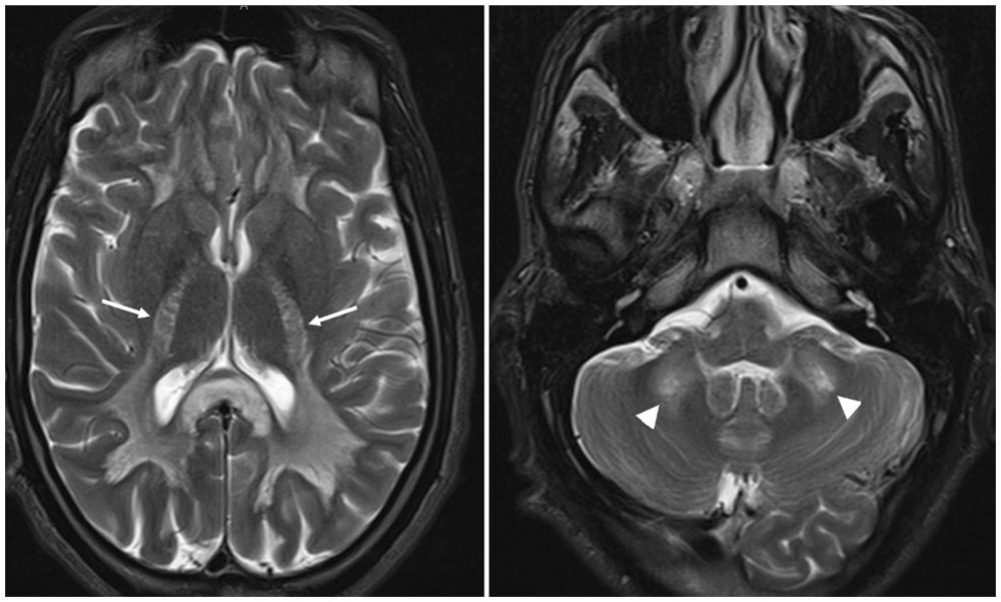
Axial T2-weighted slices passing through the thalamus and the posterior cranial fossa, demonstrate involvement of the posterior limb of the internal capsule (arrow) and the middle cerebellar peduncle sign or MCP sign (arrowhead).
